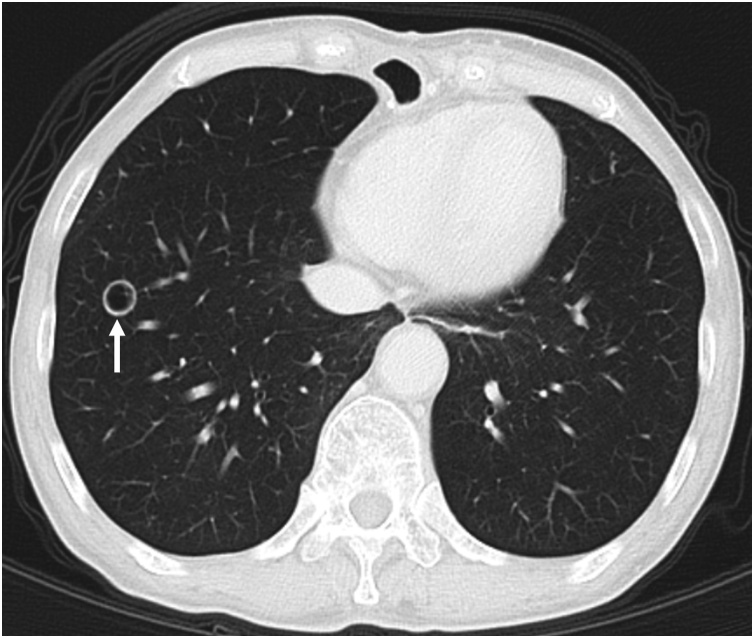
CT findings in lung window (12 months after surgery). Cavitary lesion in the lower lobe of the right lung (arrowheads).
